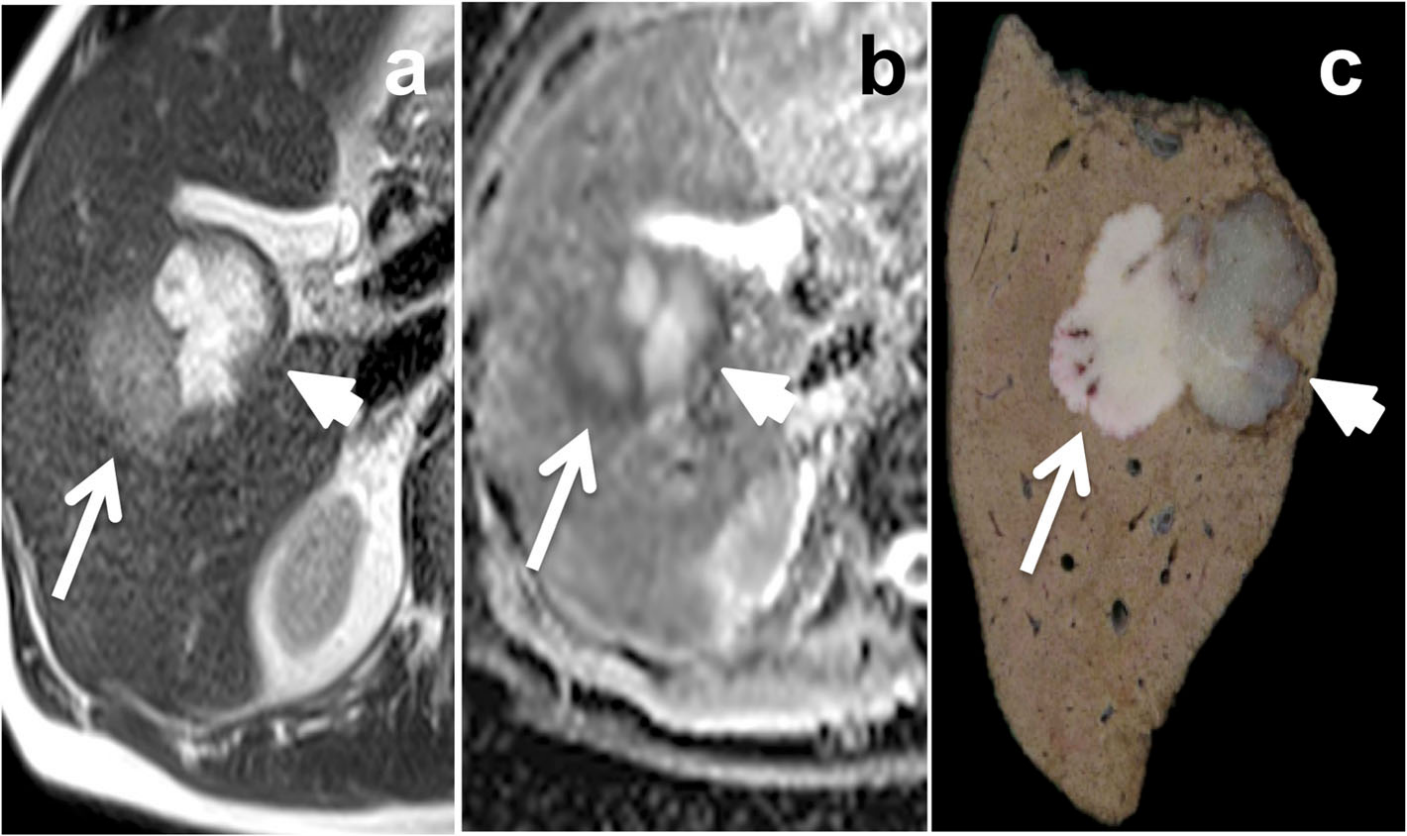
Tumor regrowth with an appearance that is different from the initial tumor in a 72-year-old male patient with mucinous right colon cancer and liver metastases. The patient received 12 cycles of FOLFOX with an objective response. Preoperative MR imaging was performed after right portal vein embolization and after discontinuation of chemotherapy for 8 weeks and showed a mucinous metastasis with a peripheral, focal, non-mucinous glandular proliferation. The initial mucinous metastasis was seen medially (arrowheads) with high signal intensity on fast spin echo T2-weighted images (a) and a central high apparent diffusion coefficient (ADC) (b). The focal regrowth was seen laterally (arrows) with mild signal intensity on fast spin echo T2-weighted image (a) and diffusion restriction with a low ADC (b). Gross pathology (c) confirmed the presence of a metastasis of mucinous adenocarcinoma (arrowhead) with non-mucinous focal regrowth (arrow)
